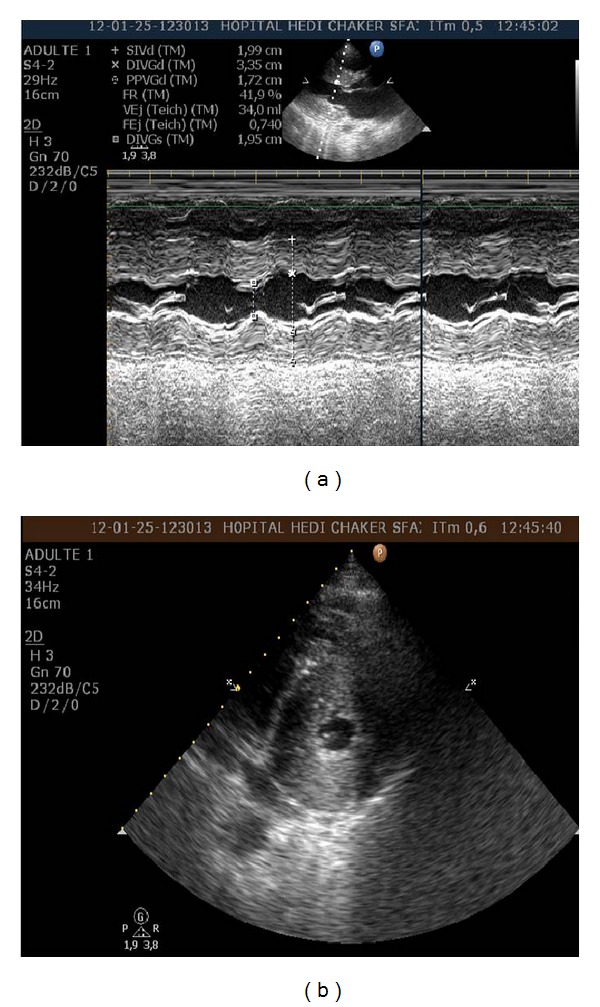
Transthoracic echocardiography revealing inappropriate left ventricular hypertrophy involving the left ventricular (LV) septum (20 mm) and the lateral wall (17 mm), midventricular obstruction.(a)Parasternal long-axis view. (b) LV apical short-axis view.
